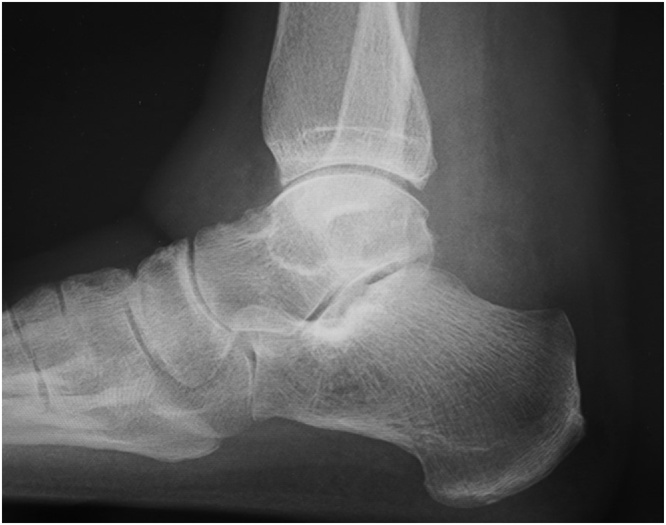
Postoperative plain radiograph acquired after surgery confirms successful resection of the enlarged posterior talar process.
